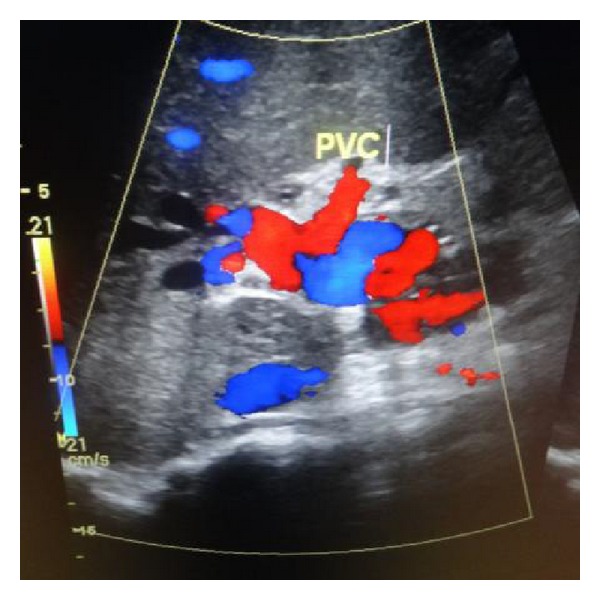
USG Doppler showing cavernous transformation of portal vein. PVC—portal vein cavernoma.
